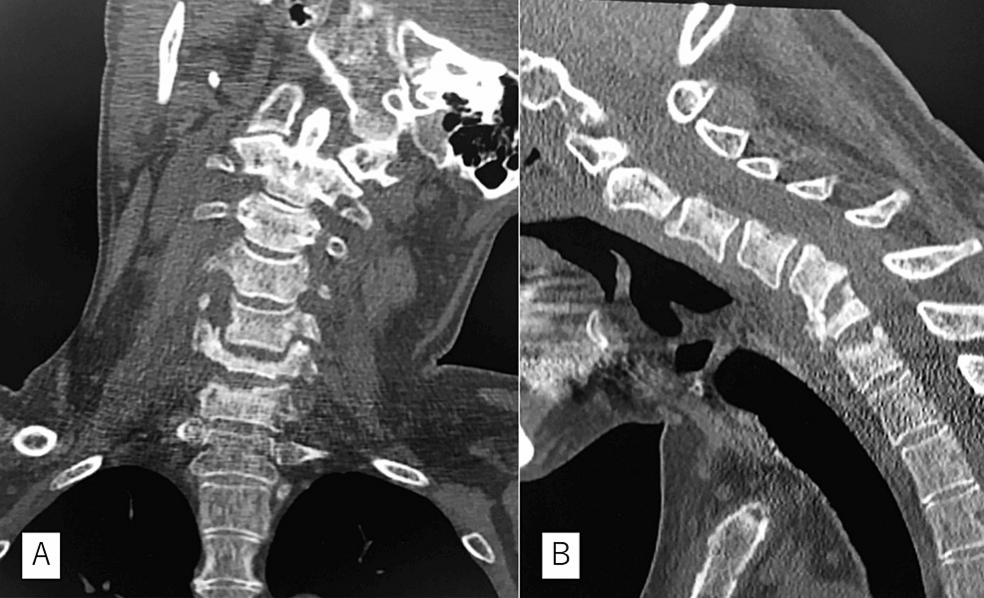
Preoperative CT scan Computed tomography revealing rotational deformity with coronal image (A) and destructive wedge deformity within sclerotic change (B).
